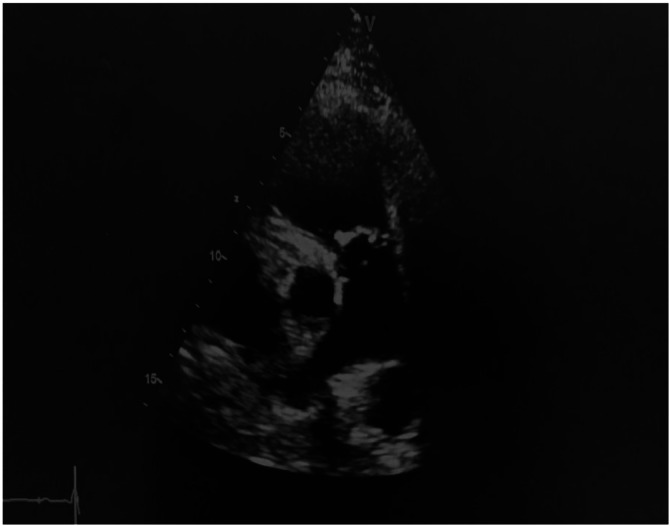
Transthoracic echocardiography at the 6-month revealed absence of the vegetations.
